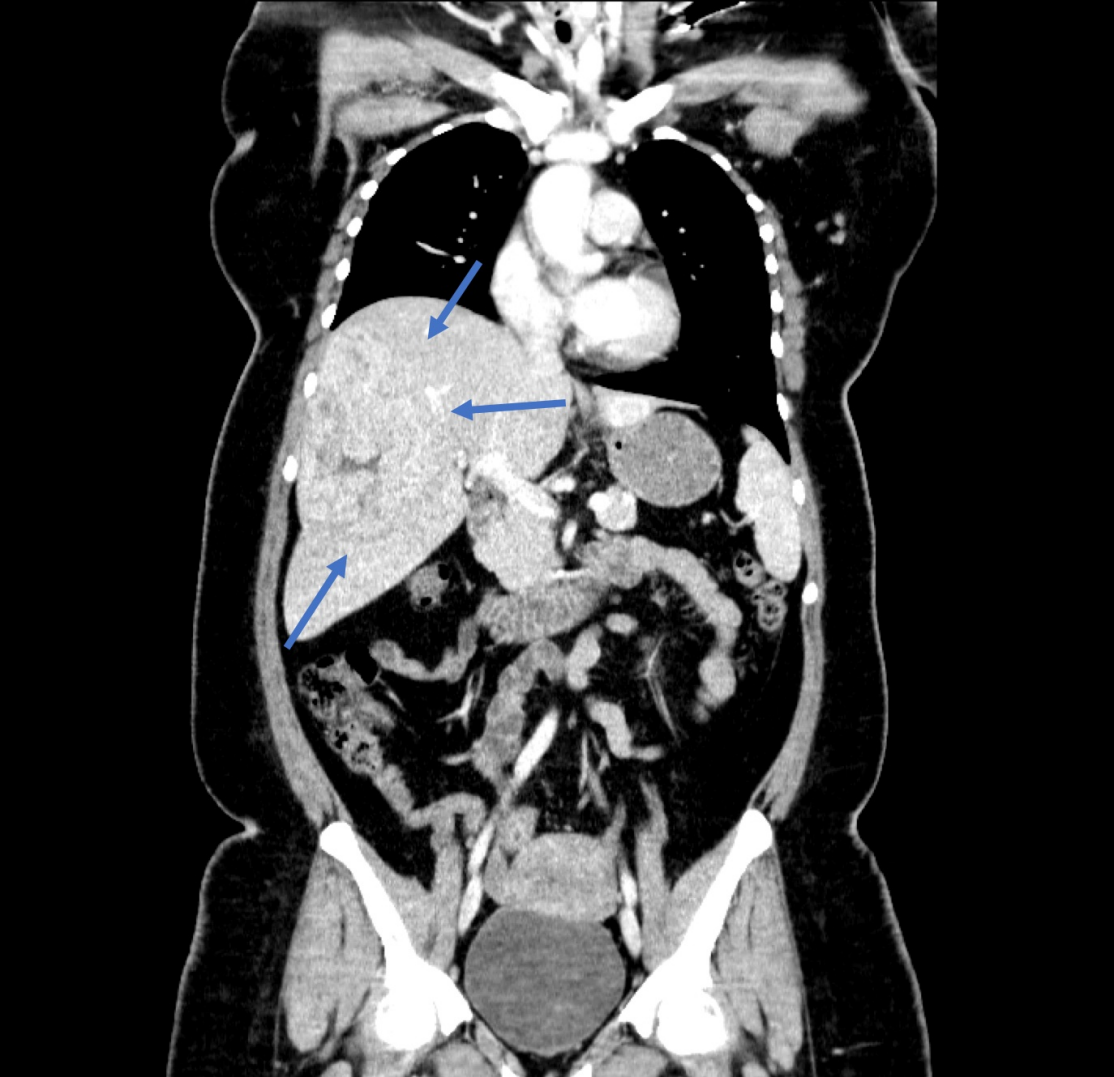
Computed tomography one year prior to presentation. Computed tomography demonstrates a 12.6 cm x 12.1 cm x 8.6 cm right liver mass with central stellate scar present, consistent with focal nodular hyperplasia (FNH). Followup with non-emergent outpatient magnetic resonance imaging (MRI) recommended.
